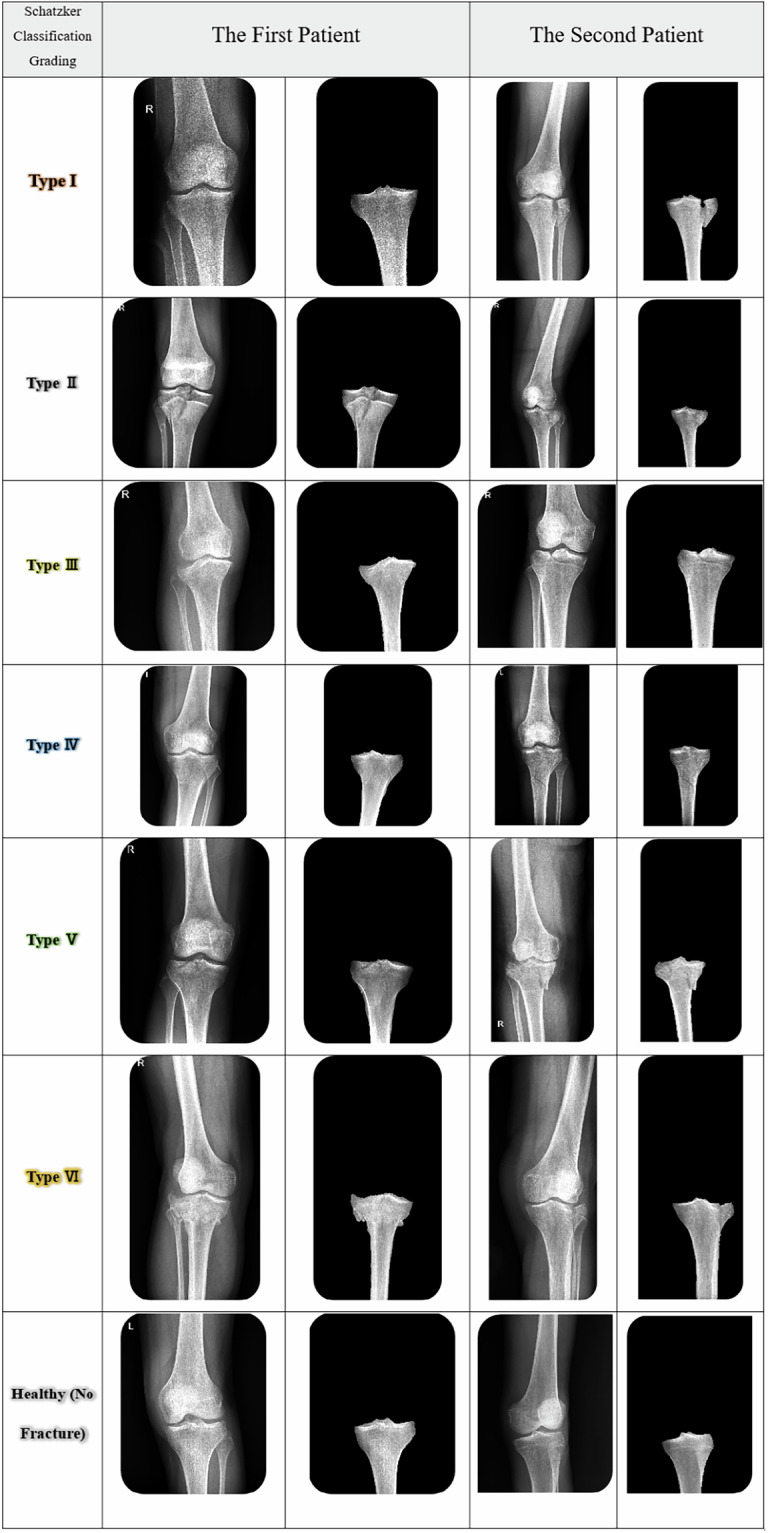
AP radiographic images of knee fractures categorized by the Schatzker classification system with tibia bone segmentation labels.
